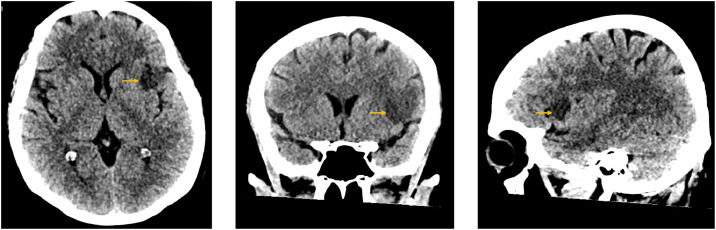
Computed tomography brain (axial, coronal and sagittal views) shows anterior aspect of the left insular cortex focal hypodensity with loss of grey-white matter differentiation and sulcal effacement, suggesting acute infarction.
